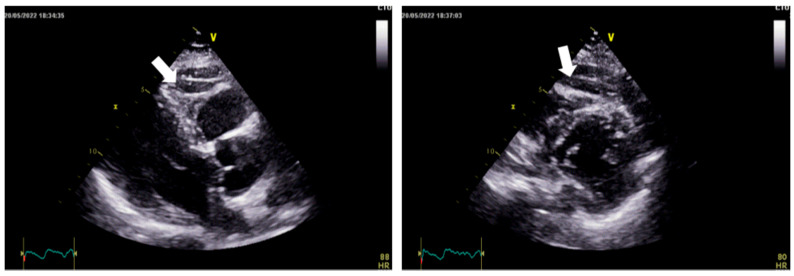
Echocardiographic assessment of epicardial adipose tissue. Parasternal long-axis views showing epicardial adipose tissue (EAT) as an echo-lucent space between the right ventricular free wall and the visceral pericardium (arrows).
